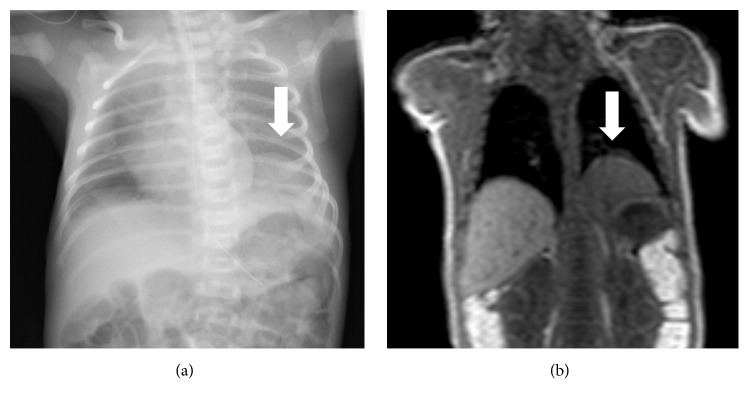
(a) A chest X-ray showed eventration of the left hemidiaphragm* (arrow)* on the first day after birth. (b) Magnetic resonance imaging revealed left sac-type CDH* (arrow)* at 21 days after birth.
